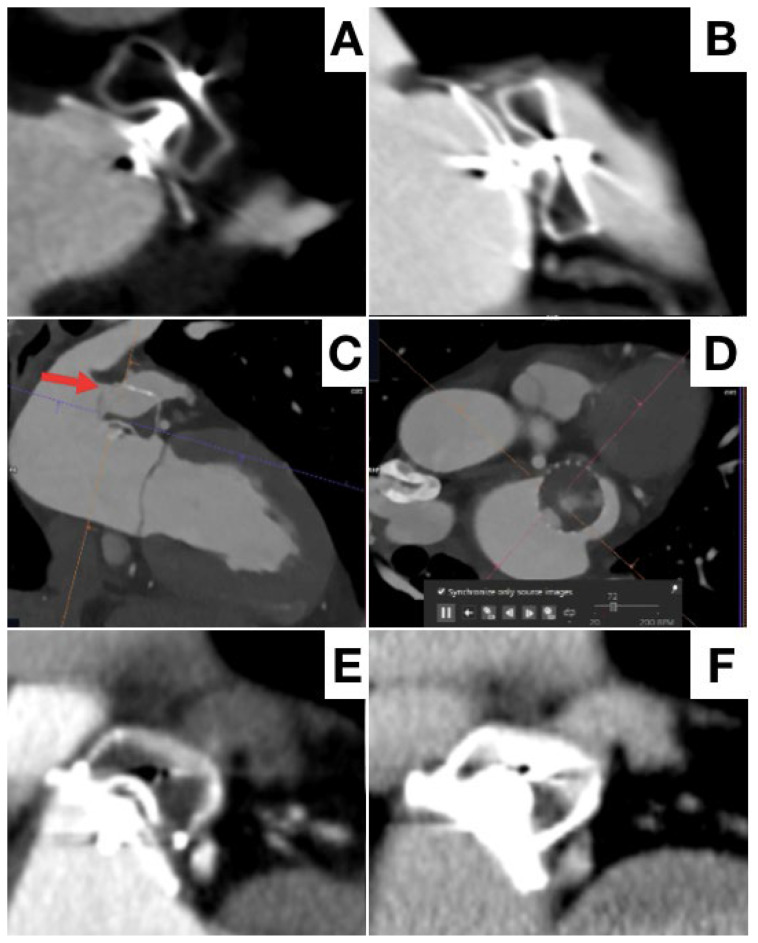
Different potential aspects of peridevice leak at follow-up by CT. No leak (A), high flow peridevice leak due to incomplete LAA occlusion (B), high flow-leak due to device malposition and rotation (C,D), low-flow leak only in venous phase (E,F).
